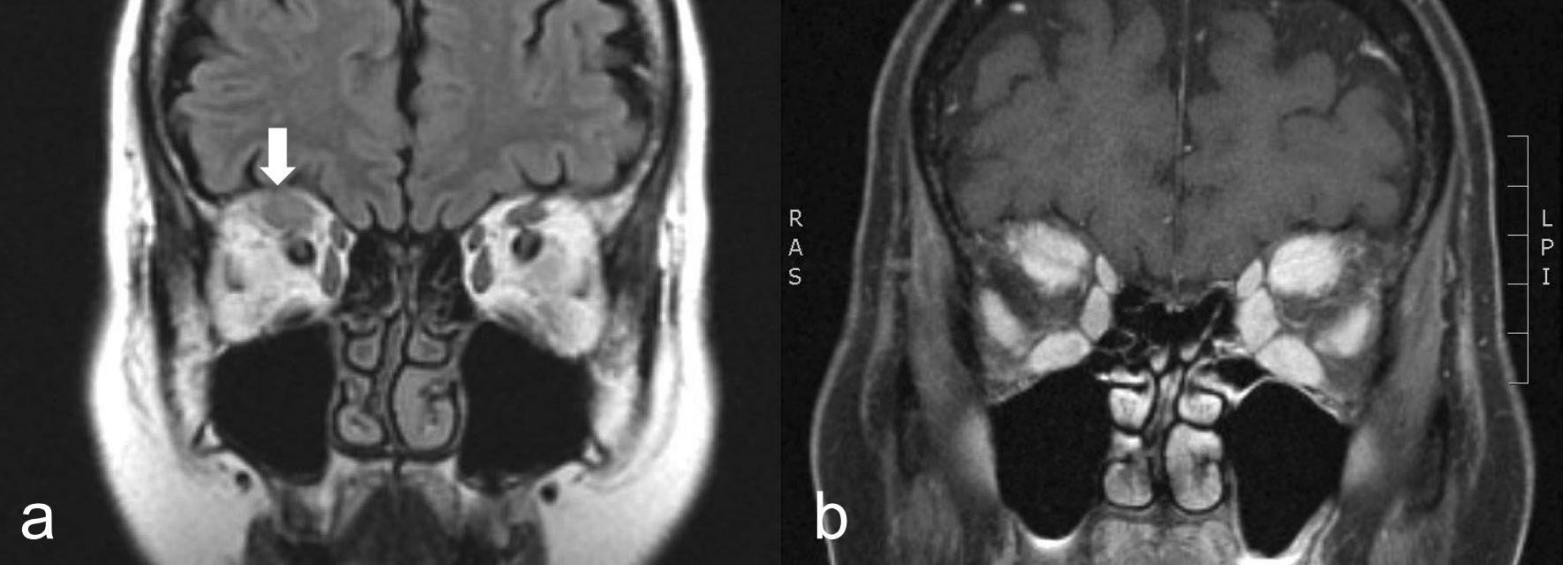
Two consecutive magnetic resonance (MR) scans of 1 patient with thyroid eye disease. (A) FLAIR MR image at initial visit shows enlarged superior rectus in the right orbit with slightly increased signal (white arrow). (B) After 8 months, fat suppressed T1-WI contrast enhanced MR of the same patient demonstrates mildly asymmetric enlargement of all extraocular muscles in both orbits with intense associated enhancement. The radiologic diagnosis for these 2 scans was non-specific orbital inflammation.
